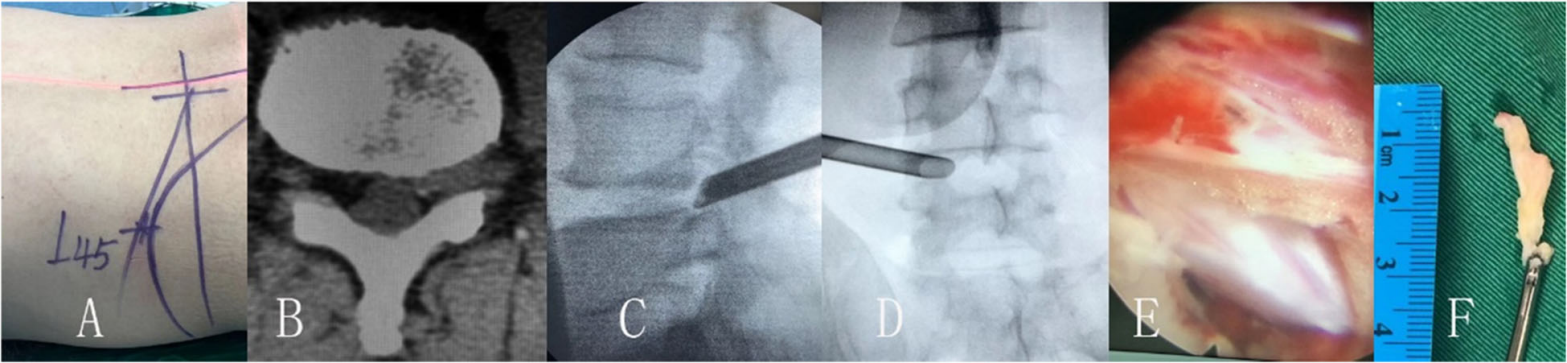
Male patient of 42 years old had L4/5 foraminal disc herniation and left leg pain combine with numbness. The leg pain was disappeared after transforaminal endoscopic lumbar discectomy and nerve decompression, but the numbness was stayed until 7 months after procedures. a show the skin marks, b was the CT image of the left foraminal disc herniation, c and d was the image of the endoscopic procedures, e show the nerve decompression, and f was the removed disc herniation fragment
